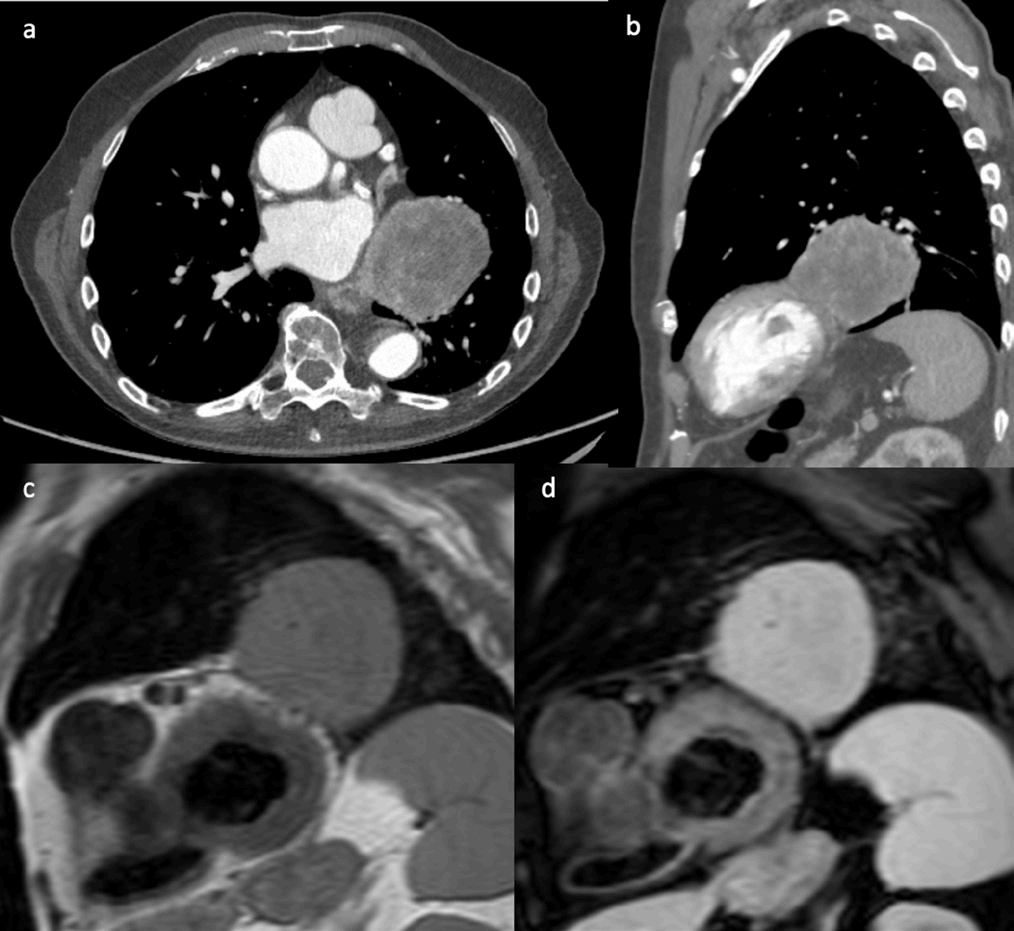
(a, b) Contrast-enhanced CT showed a large retrocardiac left lung mass
